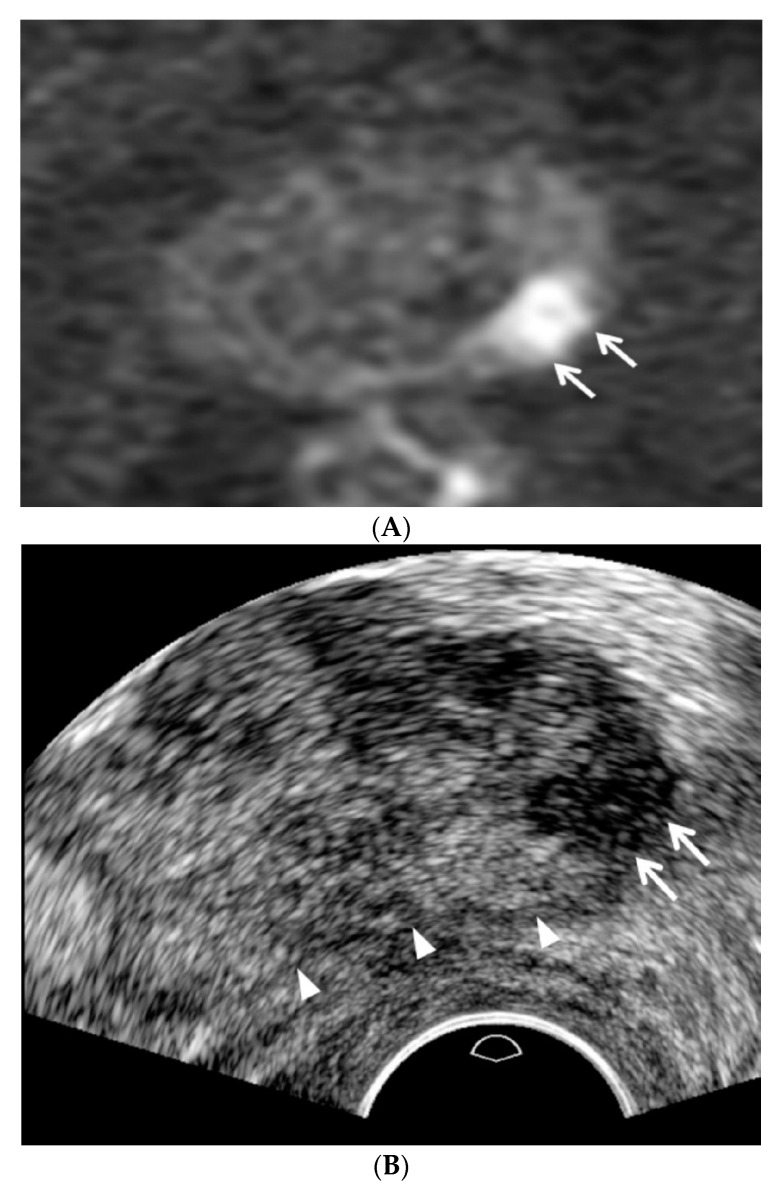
A PI-RADS 4 peripheral cancer in a 70-year-old man. (A) Diffusion-weighted axial MR image shows a hyperintense peripheral lesion (white arrows) in the left mid-gland measuring 1.3 cm, suggesting that the PI-RADS lesion category is 4. The patient’s PSA level was 3.56 ng/mL prior to biopsy. (B) TRUS axial image clearly shows a hypoechoic peripheral lesion (white arrows) in the left base. The TRUS lesion is located more superiorly to the MRI lesion. Moreover, white arrowheads indicate that the prostate is not compressed by a TRUS probe. TRUS-guided targeted biopsy alone was performed with four cores. All confirmed a Gleason score of 7 (4 + 3) adenocarcinomas.
